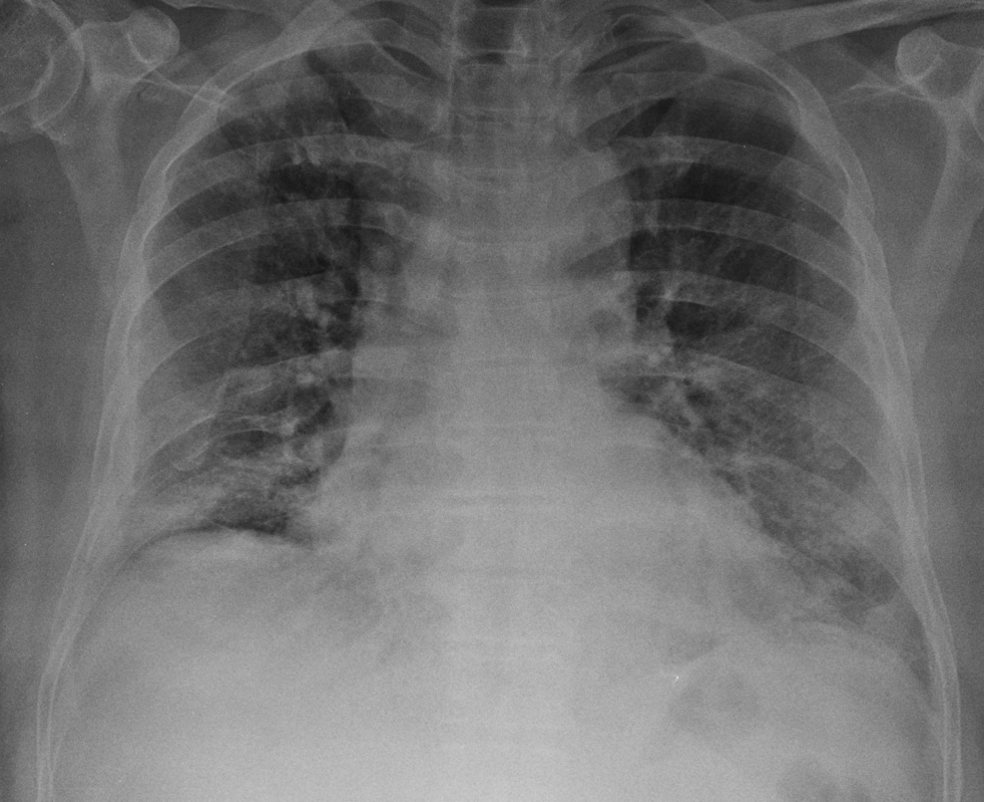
Chest X-ray of the patient on admission. Chest X-ray: peripheral patchy air space opacification in lower zones with diffuse ground opacities bilaterally.
